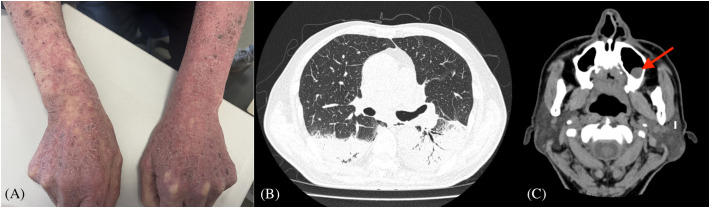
Multisystem involvement in a patient with ANCA-negative eosinophilic granulomatosis with polyangiitis (EGPA). (A) Extensive erythematous, scaly, crusted papules and desquamation over the entire body. (B) Chest high-resolution computed tomography (CT) showing bilateral patchy consolidations and interstitial changes. (C) Paranasal sinus CT demonstrating maxillary sinus mucosal thickening with small effusions(red arrow), indicating bilateral maxillary sinusitis.
